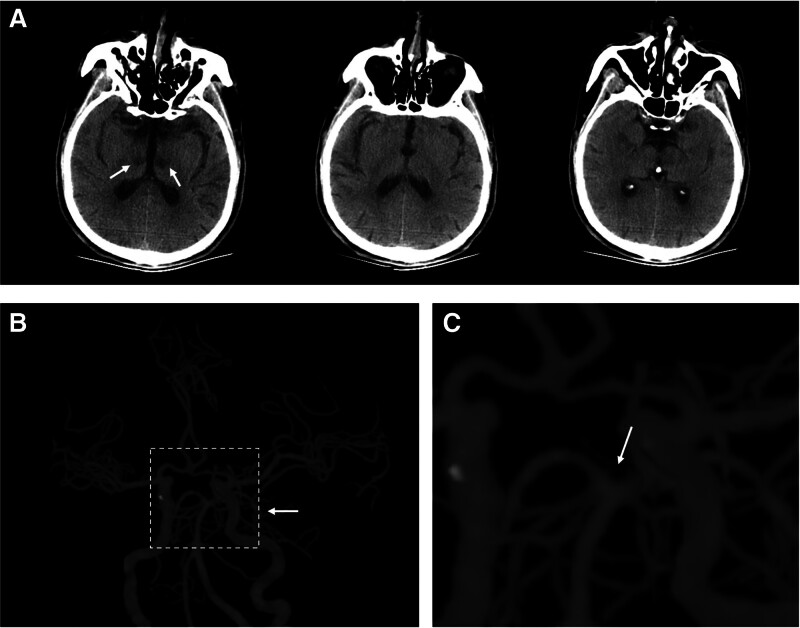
Brain imaging in the emergency room. (A) Coronal computed tomography showing bilateral hypodense lesions in thalamus (white arrows). (B) Computed tomographic angiography showing posterior cerebral artery variant, an arcade artery connecting bilateral posterior cerebral arteries, note white arrow in magnification shown in (C), and the distal of left posterior cerebral artery not clearly visualized (white arrow in B).
